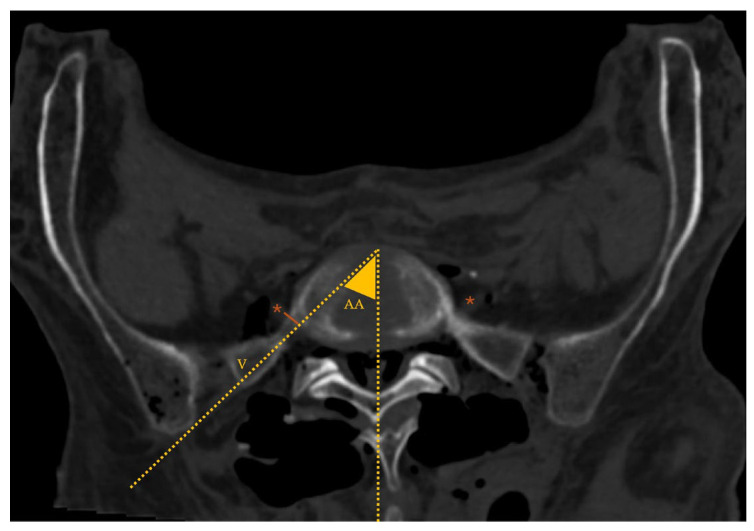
Lumbosacral spine CT—axial plane; *: ventral ramus of the fifth lumbar spinal nerve (VRL5); dotted yellow lines: marking of the approach angle (AA; angle between the midline and the vector (V) tangential to the iliac crest that crosses the most anterior point of the L5–S1 IVD space; solid orange line: minVRL5–V (minimum distance from the VRL5 to vector line).
